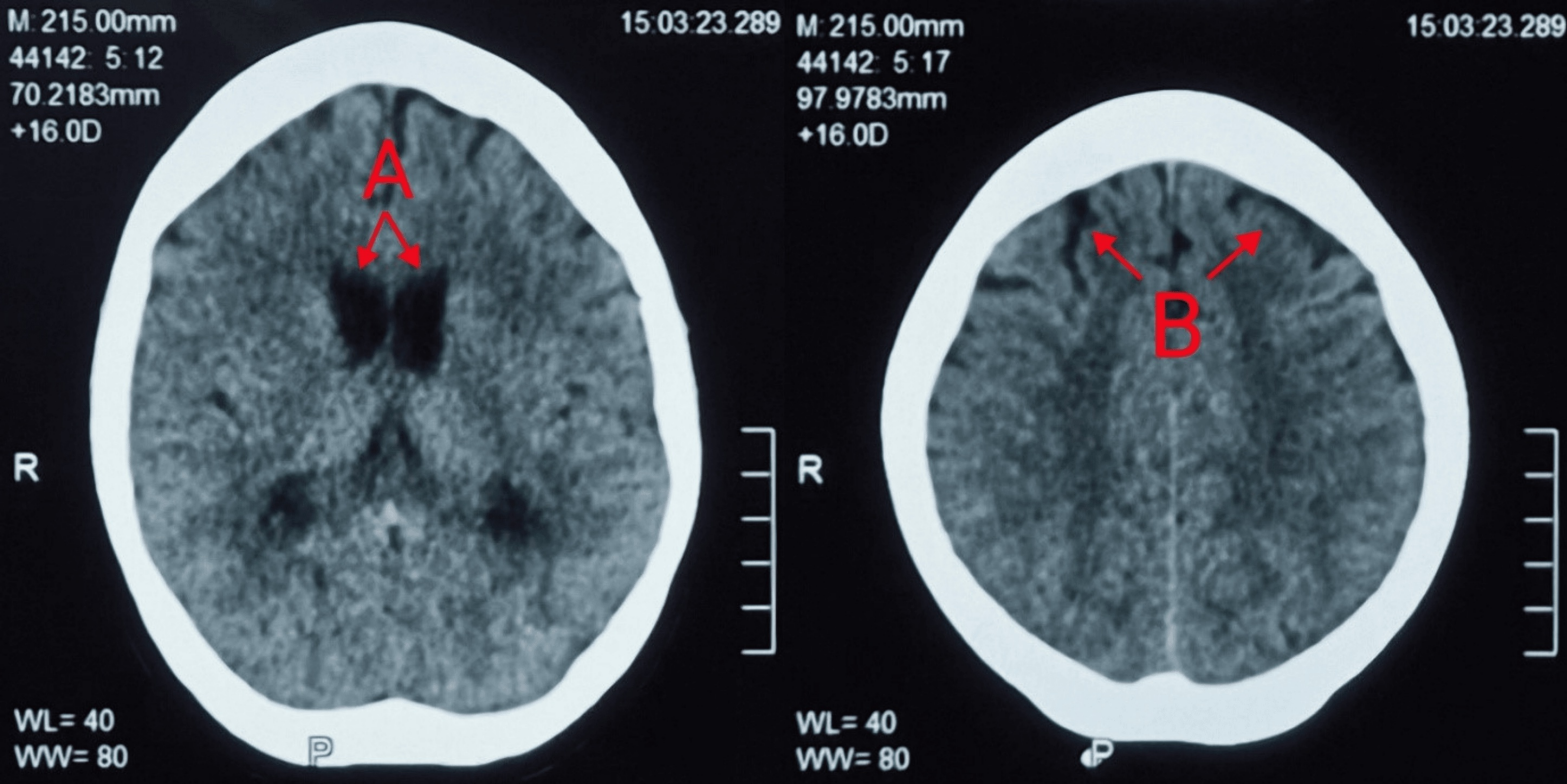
CT brain imaging revealed a marked reduction in ventricular dilatation and the resolution of previously noted periventricular hypodensities (A). The bilateral cerebral sulci are no longer effaced (B), indicating the resolution of both the communicating hydrocephalus and the associated cerebral oedema.
